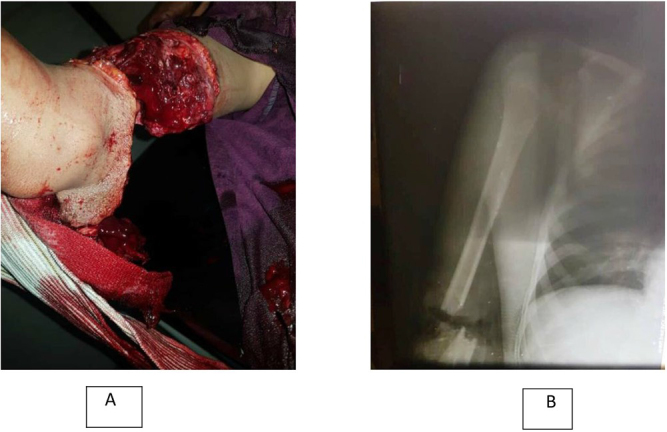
(A) The amputated part upon arrival, the arm was subtotally amputated at the lower third of the humerus, with the biceps brachii tendon remaining intact. (B) Preoperative X-ray.
